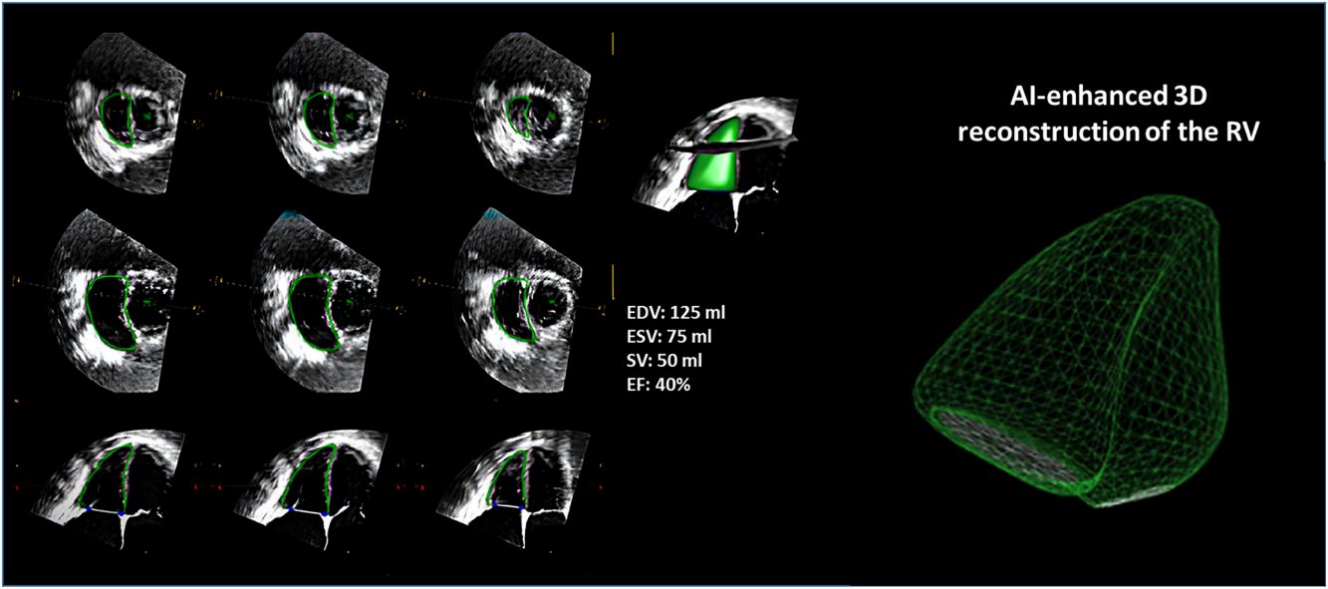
Example of AI-enhanced cardiac chamber quantification with echocardiography. The image displays multi-plane echocardiographic views of the RV with AI-enhanced automatic volumetric analyses.
